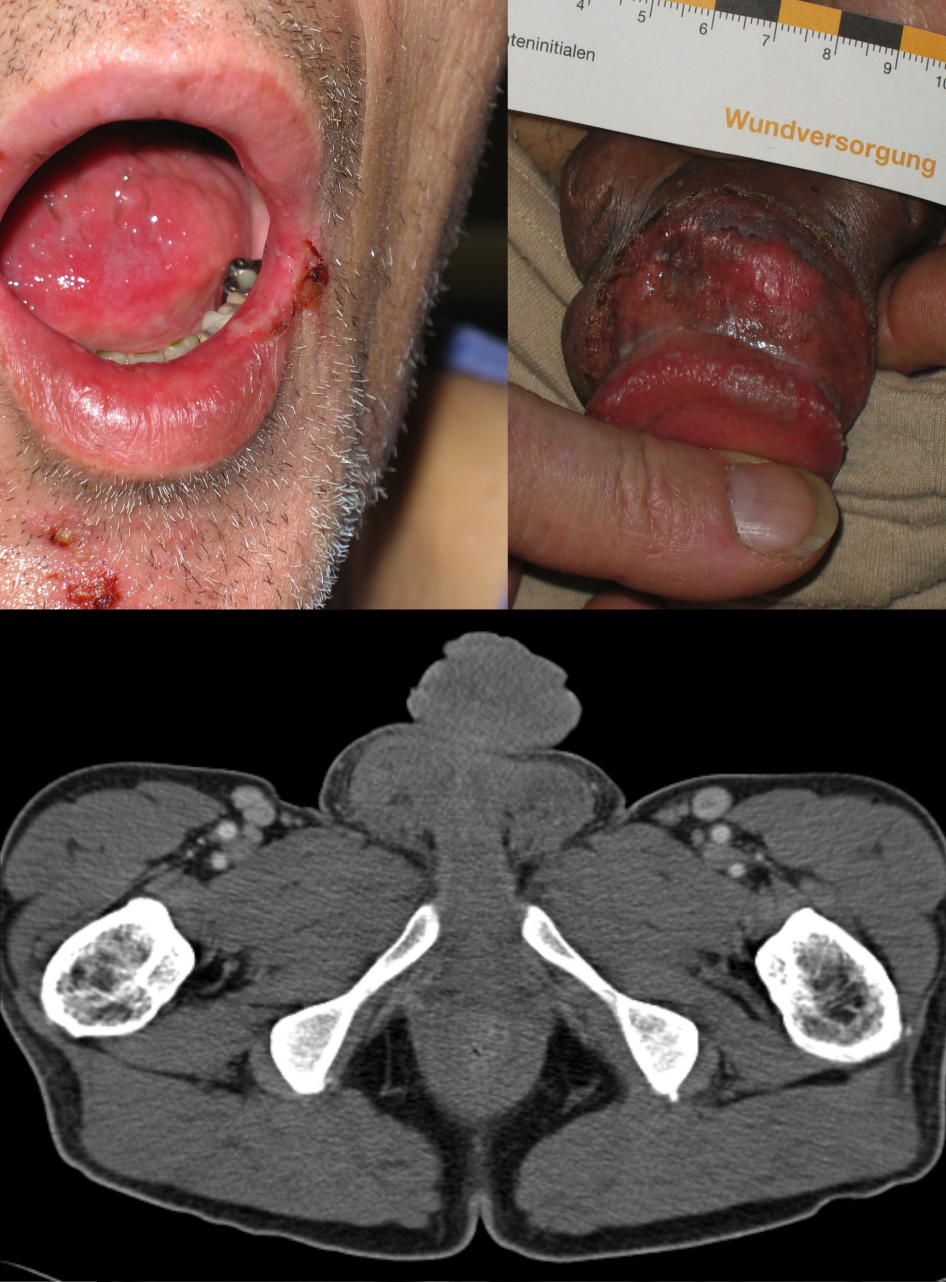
Syphilitic lesions at presentation. Upper left image: Perioral syphilitic lesion. Upper right image: Genital syphilitic lesion. PCR of smears confirmed presence of *Treponema pallidum *in both locations. Lower Image: CT scan: marked genital and scrotal oedema and inguinal lymphadenopathy.
